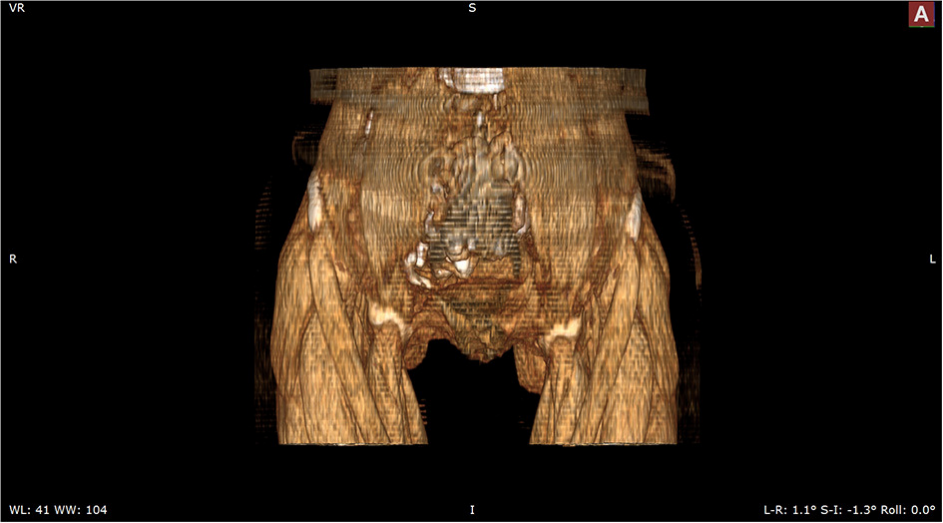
Three dimensional-computed tomography reveals the separated rectus muscles.
